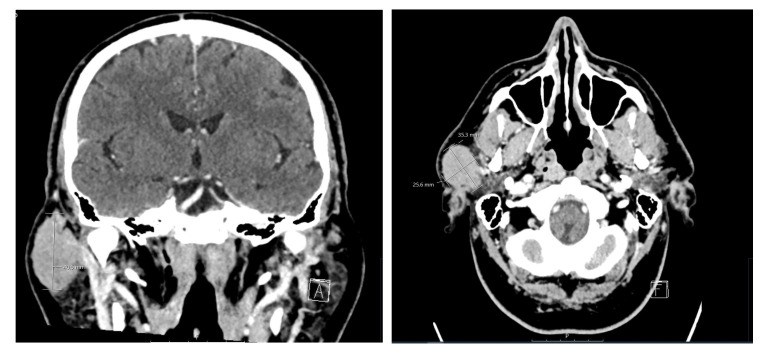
Preoperative coronal and axial views of CT imaging of the head and neck region. Soft tissue demonstrates an enhanced mass within the right parotid gland measuring at 2.5 × 3.5 cm. No enlarged lymph nodes or abdominal fluid collection can be seen.
